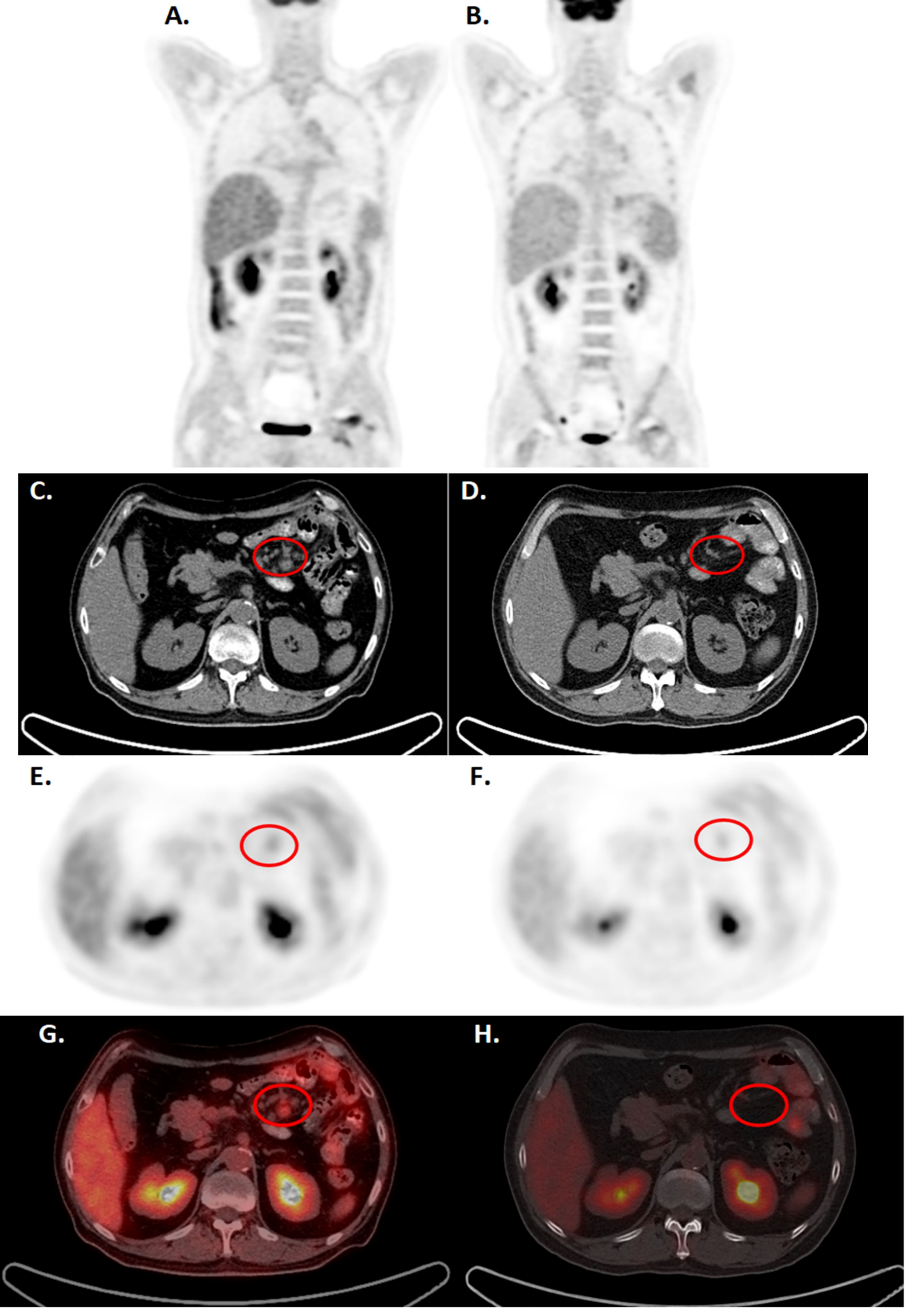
CD4-IL15/IL15sushi CAR T cells led to clinical improvements in Patient 3, diagnosed with mesenteric lymphoma, by PET-CT. (A, C, E, G) PET-CT before CD4-IL15/IL15sushi CAR T cell therapy show significant FDG uptake in mesenteric lymph nodes (circled). The larger retroperitoneal and mesenteric lymphadenopathy measure 2.4*1.7 cm, with the SUVmax of 2.1-5.2 (B, D, F, H) PET-CT 11 weeks after therapy showed reduction of mesenteric lymph node size and FDG avidity. The proto-enlarged lymph node lesions were significantly reduced or absent from before, with SUVmax of 0.9.
